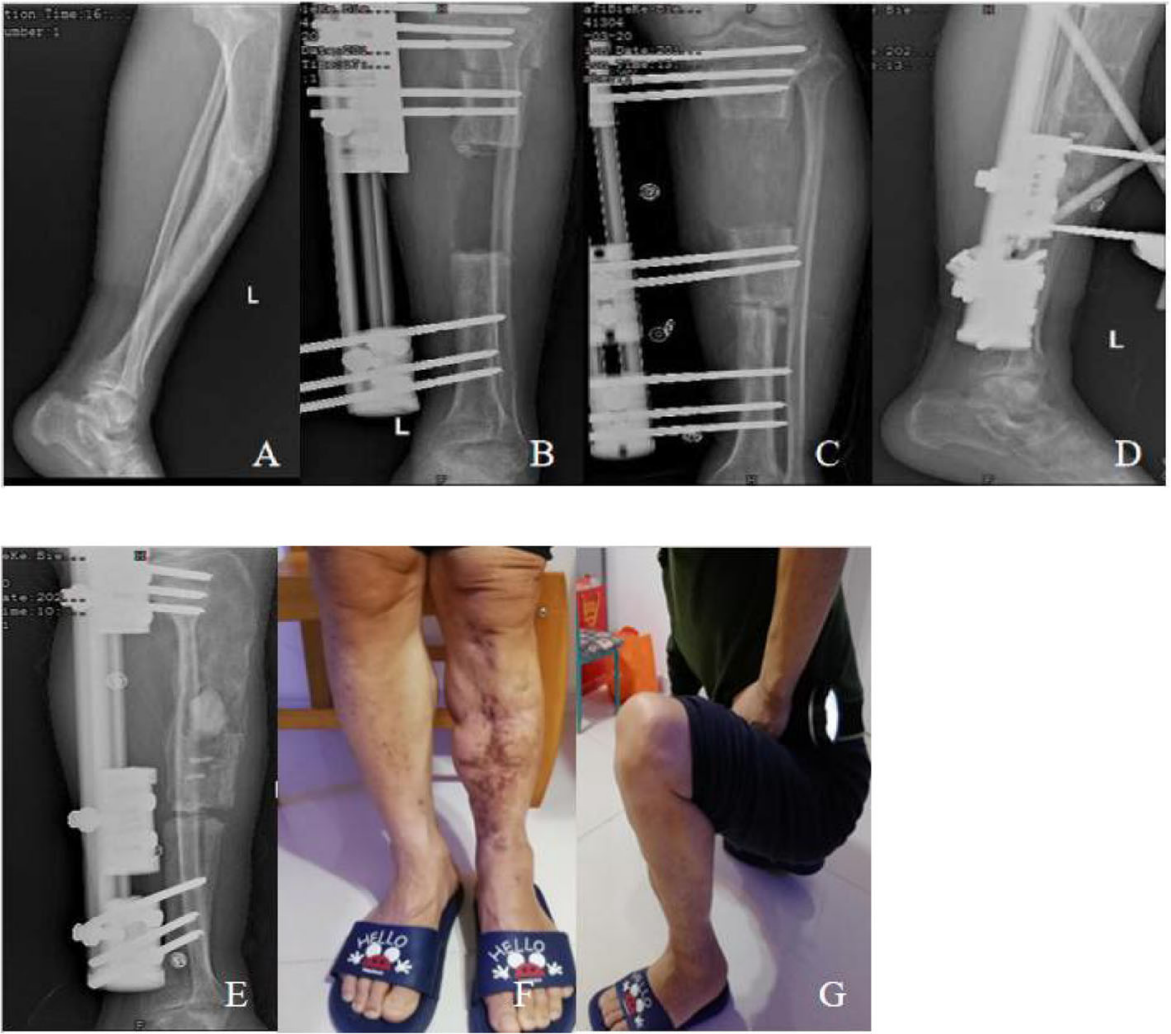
A male patient with left tibia fracture caused by traffic accident and post-traumatic osteomyelitis after plate internal fixation.(A). X-ray film at 12 months after removal of the internal fixator showing tibia osteomyelitis; (B). Anteroposterior X-ray films on the day after radical debridement and installation of external fixation; (C) Anteroposterior X-ray films at 119 days after operation, showing bone contact was reached; (D). Lateral X-ray films at 11.5 months after operation shows good regenerate consolidation,and bone grafting at the docking site.(E). After 9 months removed external fixation, infection recurrence were located in the distraction region .The patient suffered refracture at the docking site at the same time, which were treated with debridement, antibiotic cement and extenal fixation.(F,G) Function recovery at 19 months after removal of external fixator
